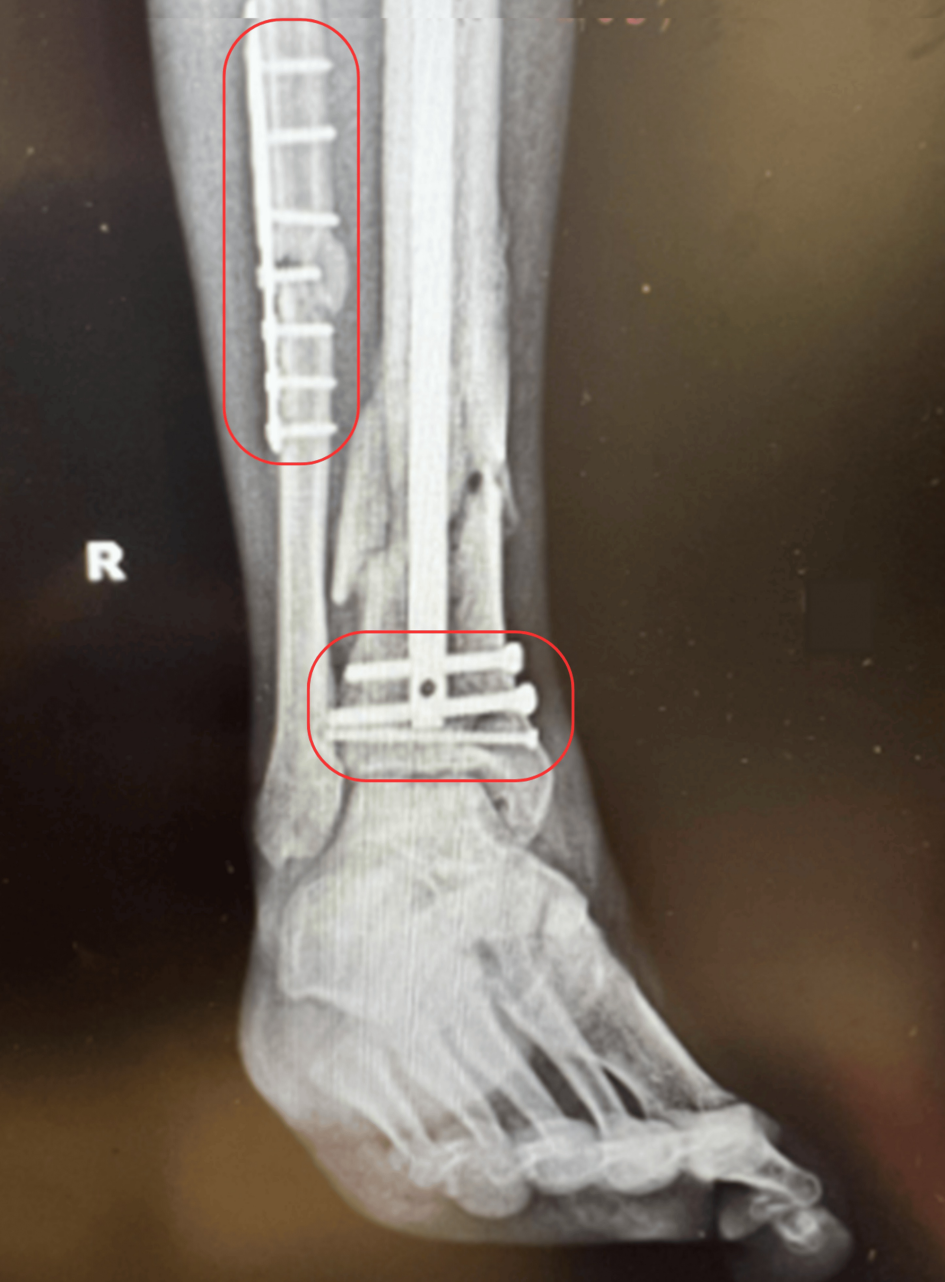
Mortise X-ray film after ORIF procedure Areas of fixation are indicated by red boxes showing good alinement post ORIF procedure. ORIF: Open reduction internal fixation
